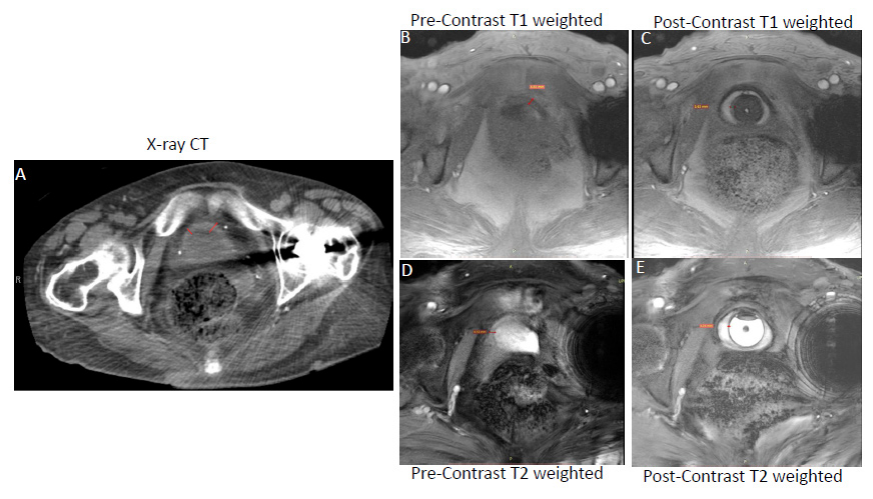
Magnetic resonance imaging (MRI) is superior to computed tomography (CT) in bladder wall segmentation. Contrast-enhanced T
